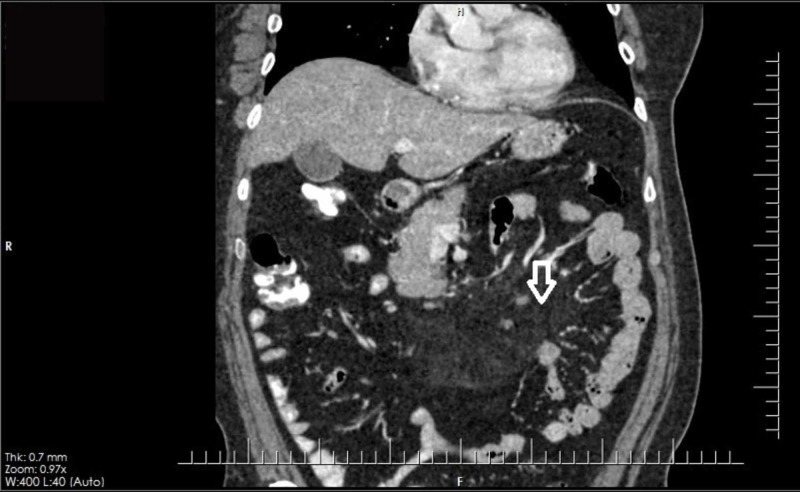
CT coronal view showing ill-defined, mesenteric fat stranding in the center of the abdomen – in the umbilical and supraumbilical locations and encasing the vessels
